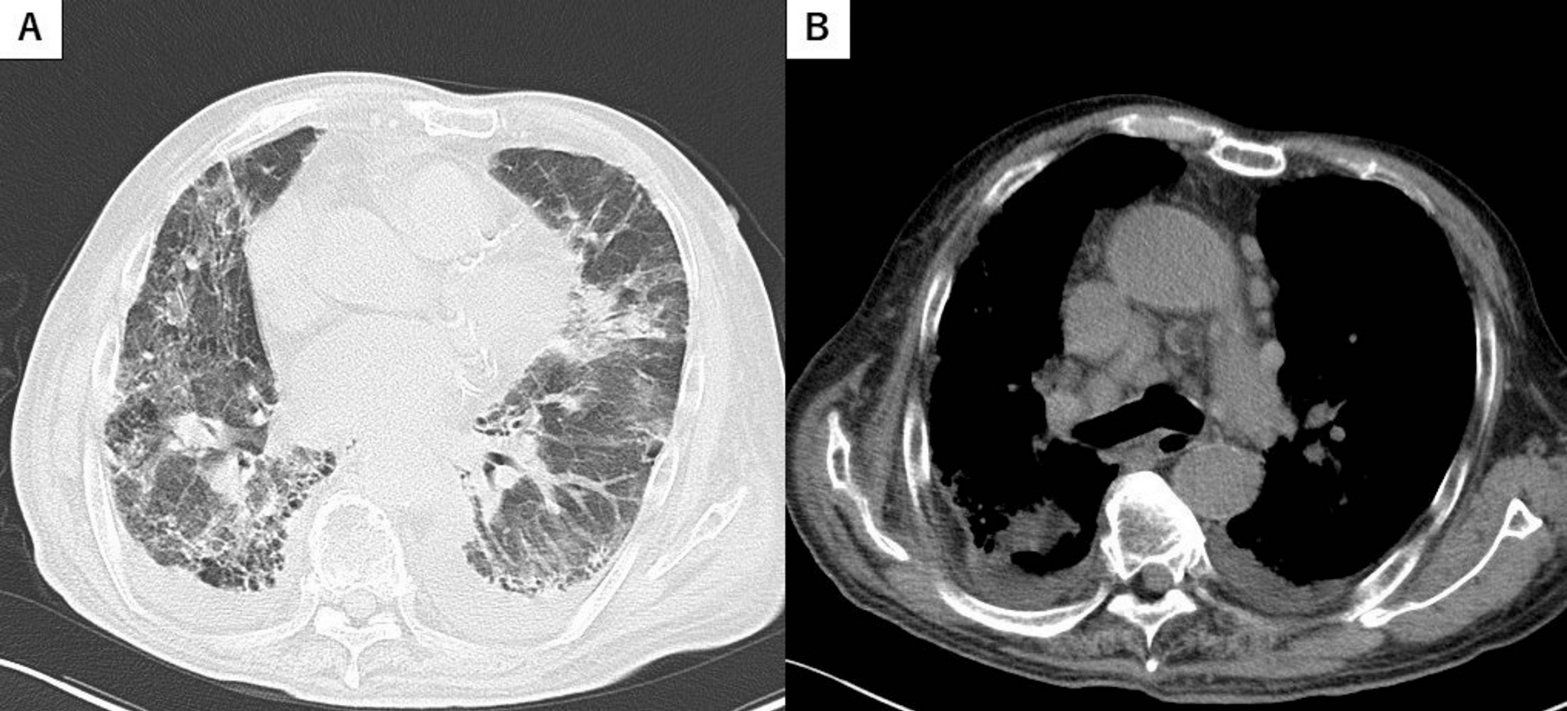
Computed tomography on admission of case 2. (A) Lung window image shows peripheral ground-glass reticular opacities, traction bronchiectasis, and cystic changes in both lungs, along with consolidation and pleural effusions in the upper and lower lobes. (B) Mediastinal window image reveals multiple enlarged mediastinal lymph nodes, including in the pretracheal and subaortic regions.
